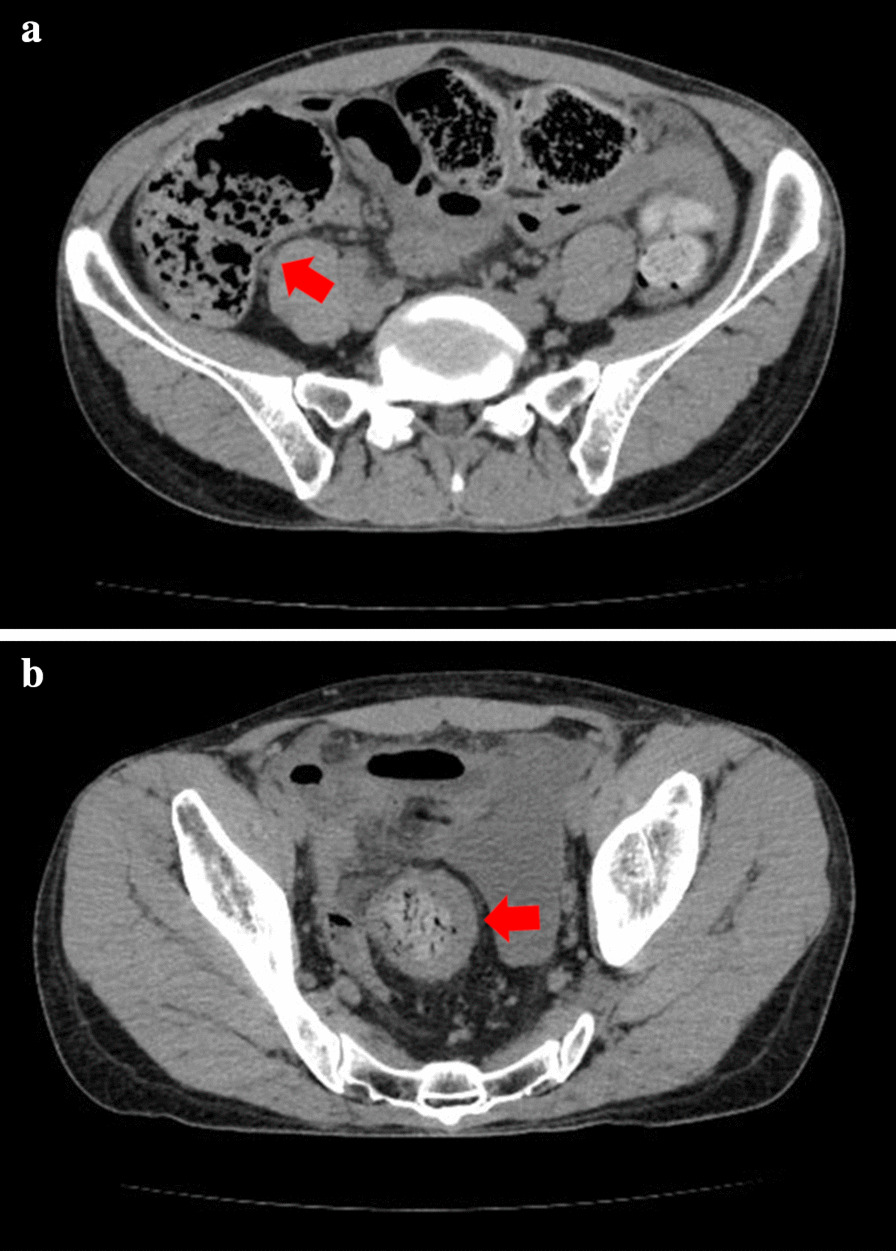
Preoperative CT findings. Abdominal CT scan showing intraperitoneal free air and colon distension, suspicious of gastrointestinal perforation (a). Pelvic CT scan showing the perforation site in the rectum but does not clearly reveal the presence of the lymphoma (b)
